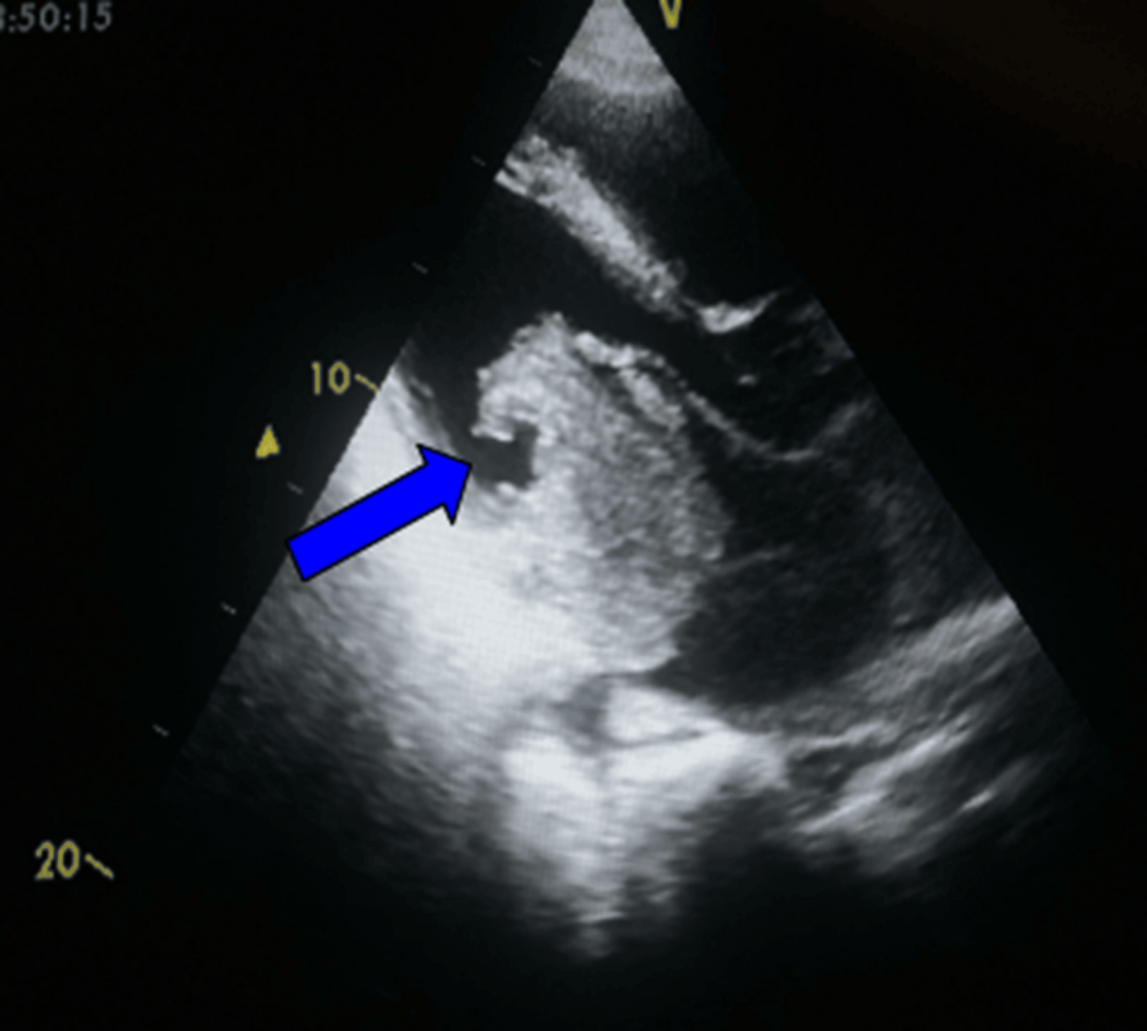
Transthoracic echocardiography (parasternal long-axis view) showing a mobile mass in the left atrium prolapsing through the mitral valve during diastole (arrow)
